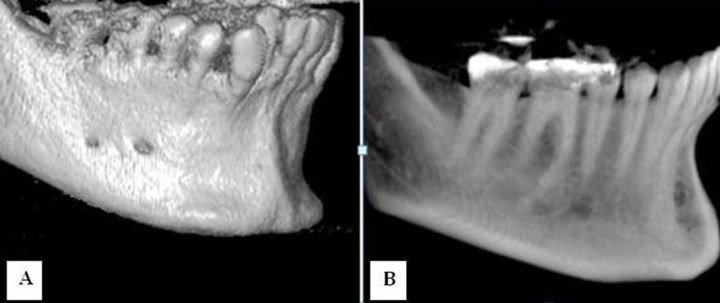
CBCT images show the mental foramen and accessory mental foramen below the apex of second premolar and first molar, respectively; *A*) volume style and *B*) ray cast style
